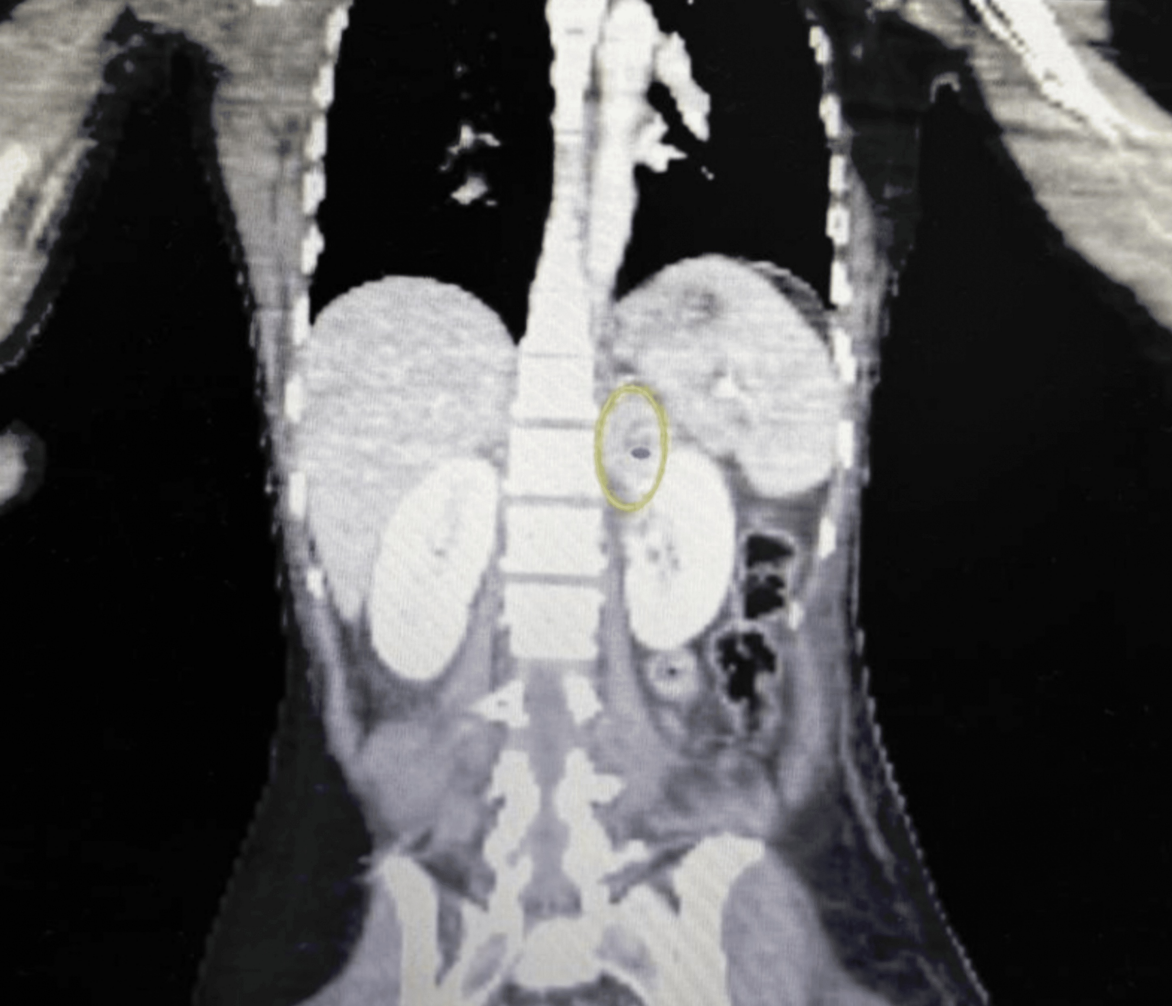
CT angiography showing a 4 × 4 cm left adrenal mass with peripheral arterial phase enhancement (yellow oval) and a central heterogeneous cystic component.
